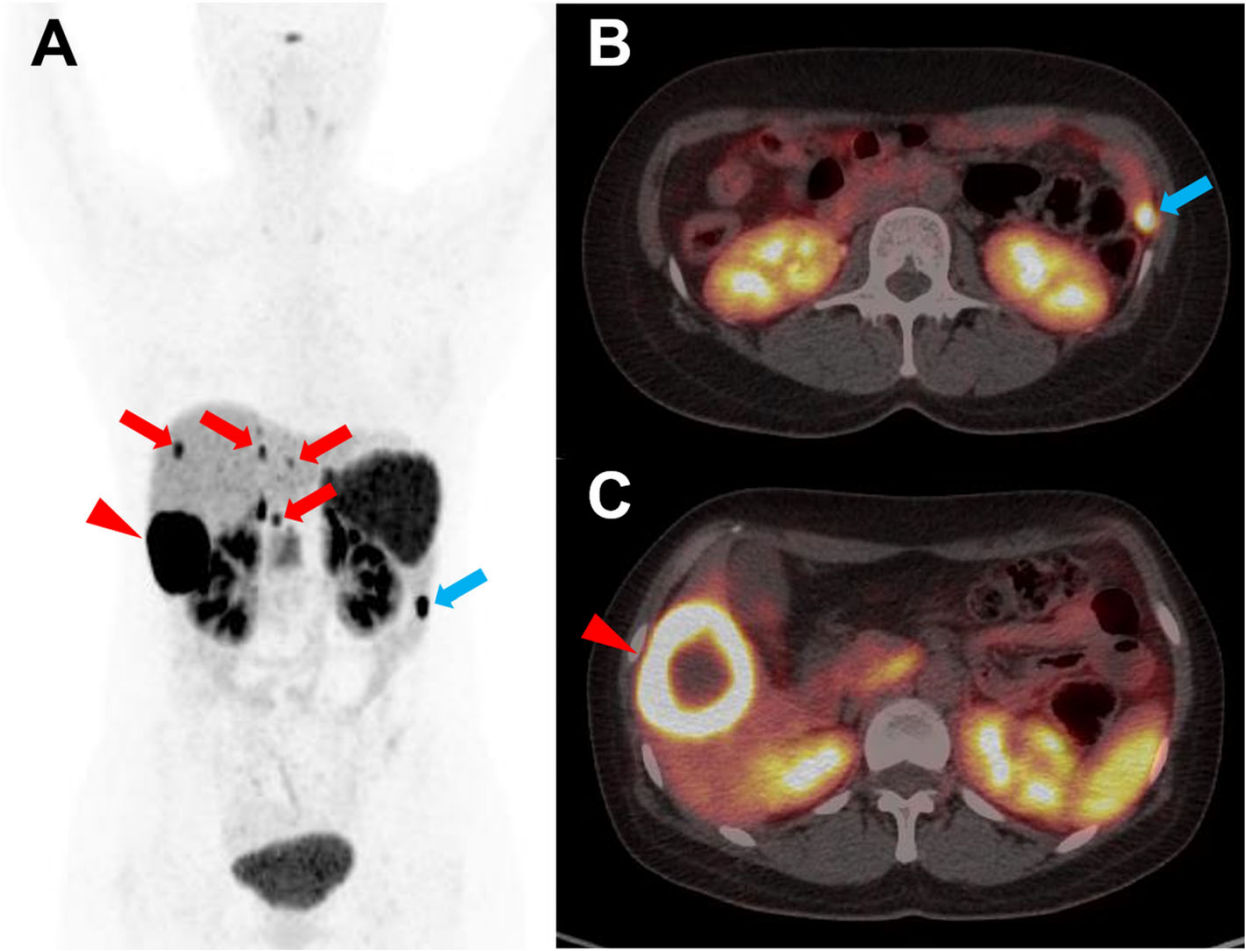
Representative case of a 37-year-old male with a primary jejunal NET grade 2. MIP (a) and trans-axial (b, c) [^68^Ga]Ga-DOTA-TOC PET/CT images reveal primary NET at the jejunal loop (blue arrow) and multiple small (red arrows) and large (red arrowhead) hepatic metastases with intense uptake. He had high TLR (17.7) and high SUVmax (77.5), predicting a good prognosis. However, he had a poor ECOG performance status (1) and high WTV (112.5), predicting poor prognosis. After lanreotide therapy, no progression was found until 30 months of follow-up
